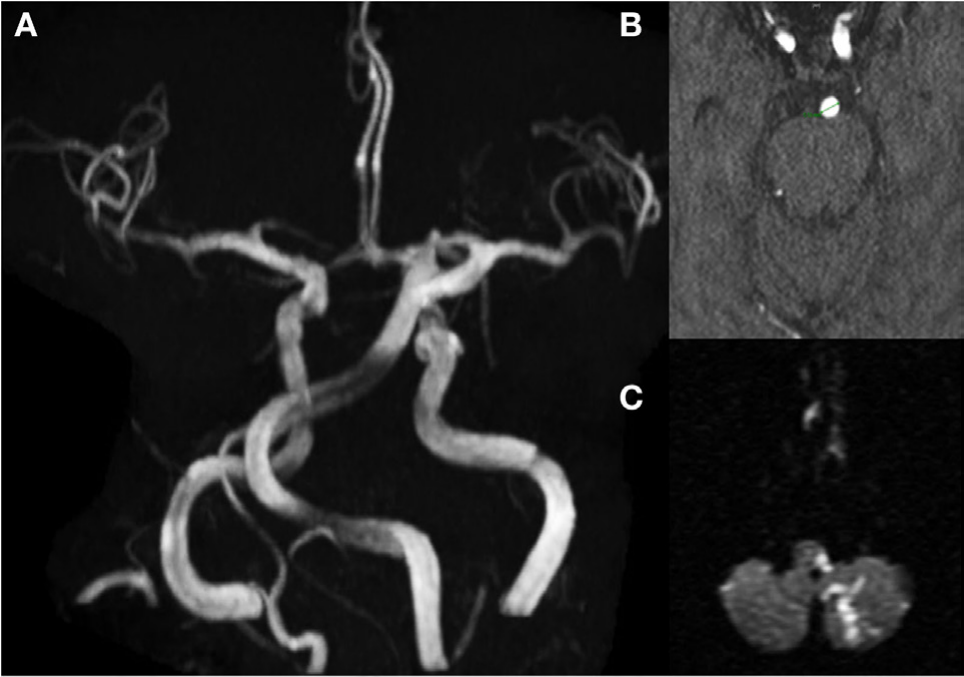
MRI/A brain of a 67-year-old man presenting with left lateral medullary syndrome, and left posterior inferior cerebellar artery (PICA) territory infarct; (A) MRA brain showing elongated and tortuous basilar artery (BA); (B) axial cut showing increased BA diameters (5.5 mm); (C) diffusion-weight restricted lesion on left PICA territory.
